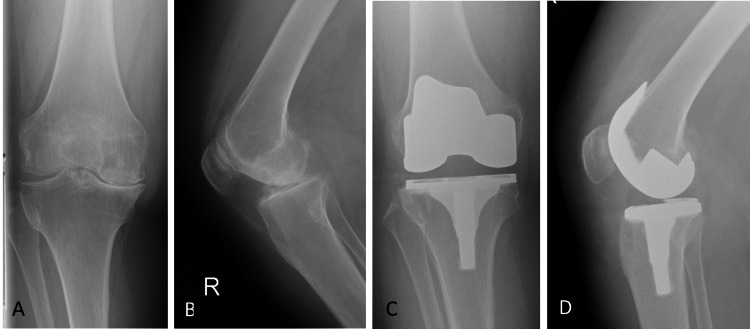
Radiographic images of the right knee. (A and B) pre-operative AP and lateral images showing end-stage knee OA. (C and D) showing post-operative AP and lateral images with well-position implants. OA: Osteoarthritis; AP: Anteroposterior
